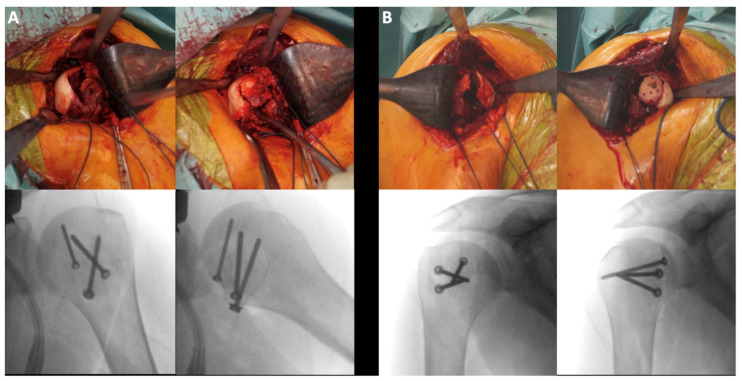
33-year old male patient after an epileptic seizure: bilateral posteriorly dislocated impression type fractures of the proximal humerus; (A) intraoperative pictures and X-rays of the left proximal humerus: open reduction, allo- and autograft, fixation with three screws (3.5 mm + 2.7 mm); (B) intraoperative pictures and X-rays of the right proximal humerus: open reduction, allo- and autograft, fixation with three screws (3.5 mm + 2.7 mm).
